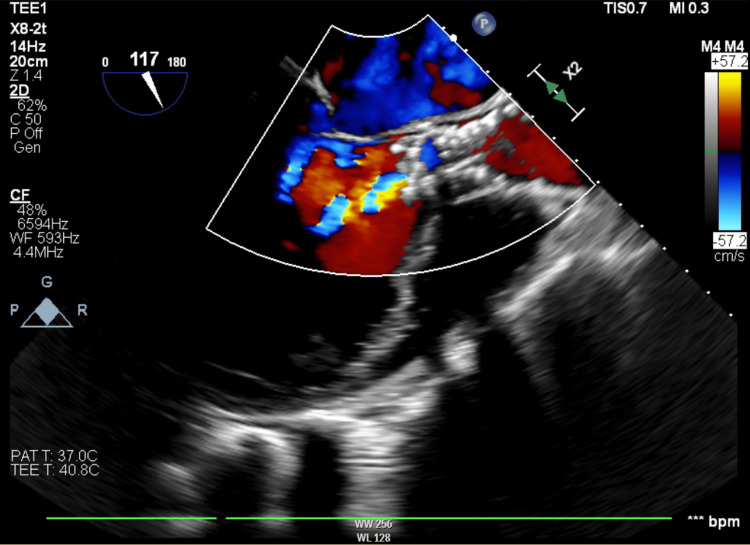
Intraprocedural TEE of the aortic valve with color Doppler, mid-esophageal long-axis view, demonstrating severe prosthetic valvular regurgitation as a result of the infolding TEE: transesophageal echocardiography
